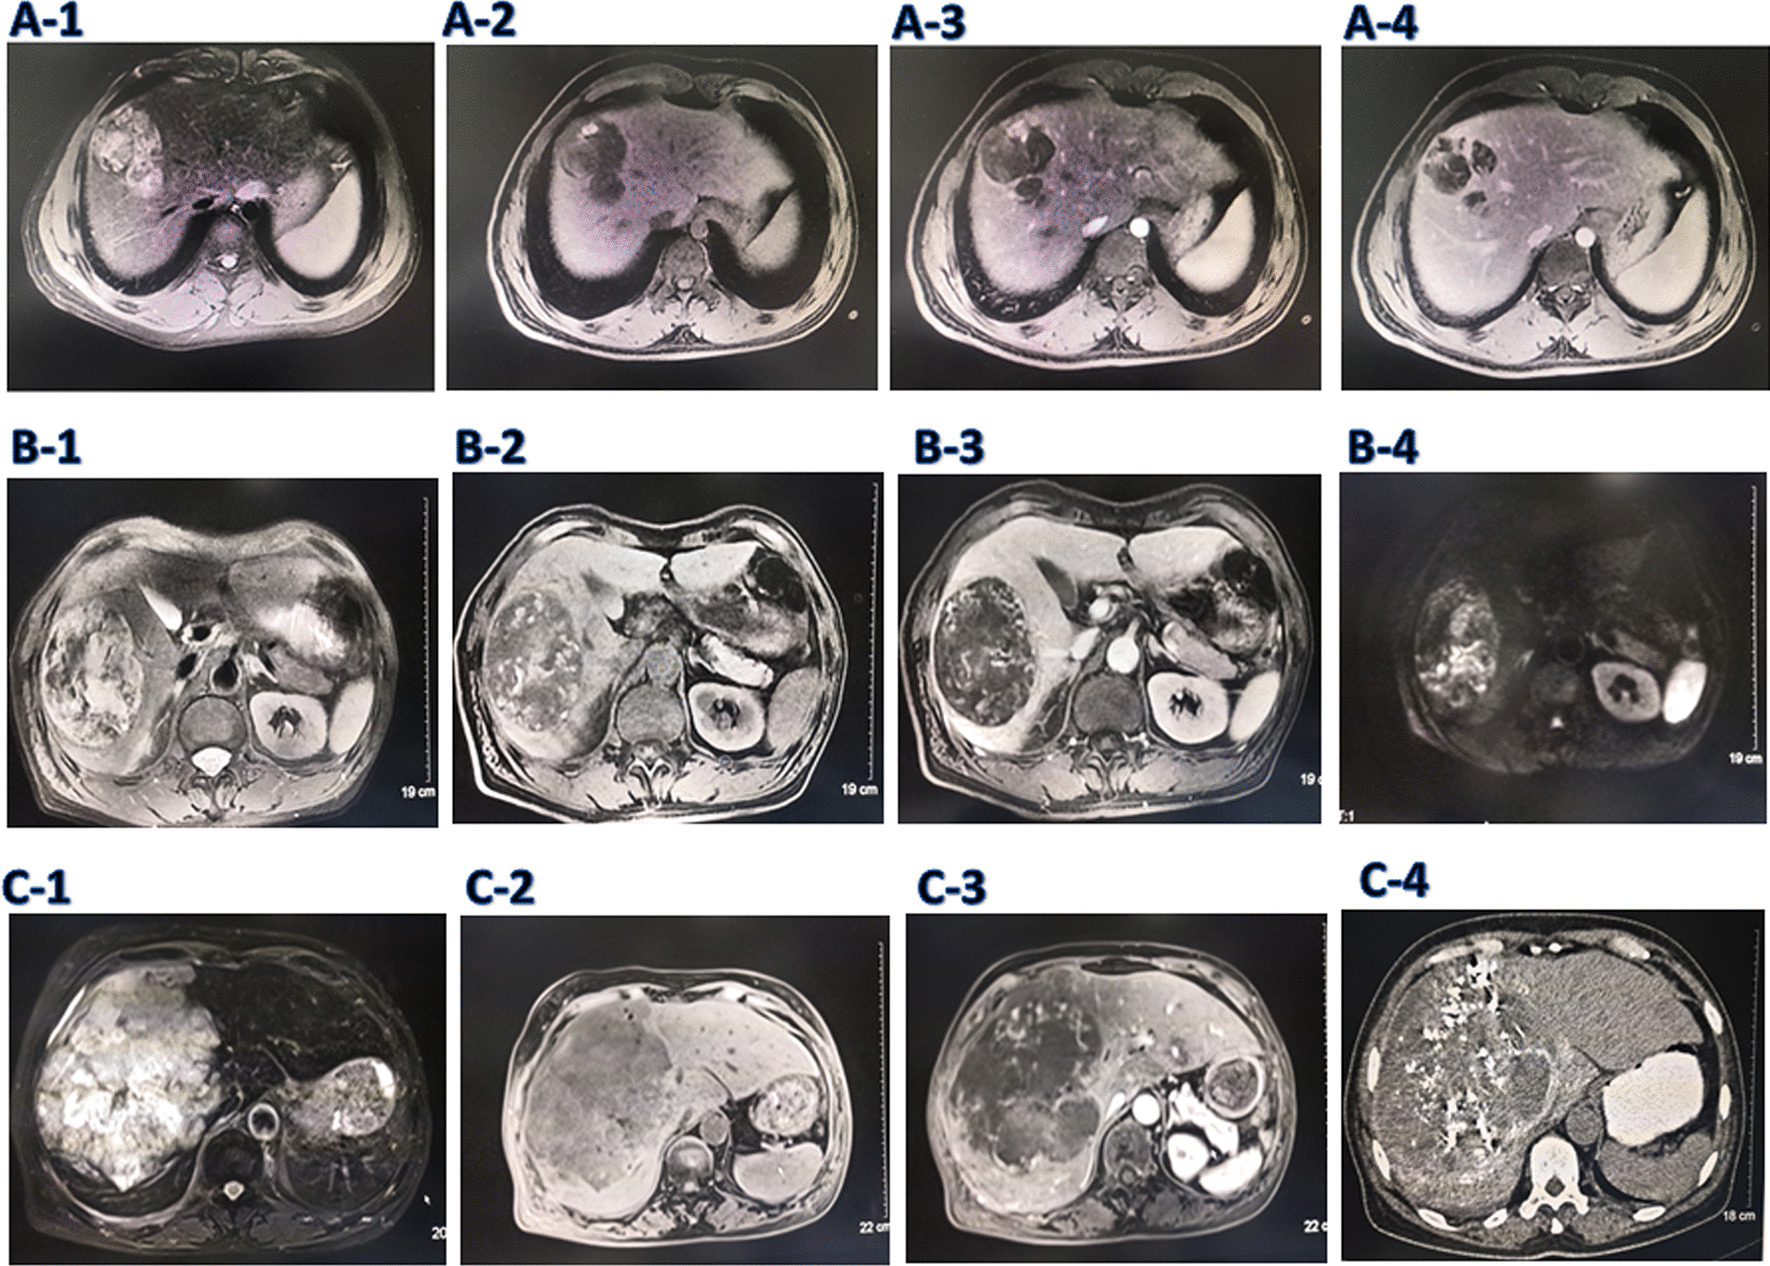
The imaging findings of PHA patients 1, 2 and 3. Patient 1. An irregular space occupying lesion with a size of 5.7 × 4.3 × 4.3 cm could be seen in the right liver. *A-1*. On T2WI, there was a high signal, septum and mixed signal shadow; *A-2*. On T1WI, most of them were hypointense, and a high signal nodular shadow was seen on the edge; *A-3*. On the contrast-enhanced scan, there was a little nodular enhancement; *A-4*. In portal phase, the enhancement of marginal nodule disappeared, and the septal stroma gradually strengthened. Patient 2. A tumor about 6.7 × 8.8 × 8.0 cm in size was found in the right liver. *B-1*. On T2WI, there was mixed high and low signal intensity; *B-2*. On T1WI, most of the tumors were hypointense and a few were patchy hyperintense; *B-3.* Irregular strip and progressive enhancement were found in the tumor. *B-4*. MR diffusion weighted imaging showed that most of the tumors showed mixed restricted diffusion. Patient 3. A tumor about 15 × 14 × 13 cm in size in the whole right liver, part of which was located in the left liver. *C-1*. On T2WI, most of the tumors showed high signal intensity, a few of them were extremely high signal, and a small amount of low signal. *C-2*. On T1WI, the tumor showed hypointensity. *C-3.* On a contrast-enhanced scan, the tumor showed irregular strip reinforcement. *C-4*. One month after TACE, a CT scan of the liver showed that there was a lot of lipiodol accumulation and deposition in hepatic angiosarcoma
